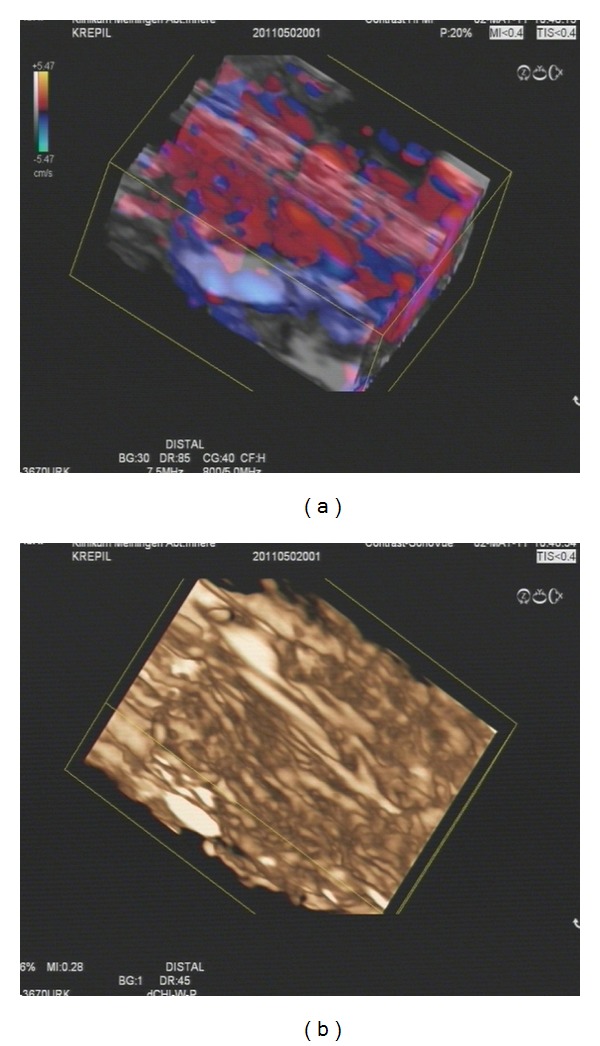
Contrast-enhanced high and low mechanical index endoscopic ultrasound with 3D reconstruction: (a) dense vessel involvement of the pancreas is impressively visible; all the red and blue spots are the Doppler colour signals from a section of the pancreas; (b) the influx of contrast enhancer is shown clearly in the low MI reconstruction to have spread homogenously through the organ.
